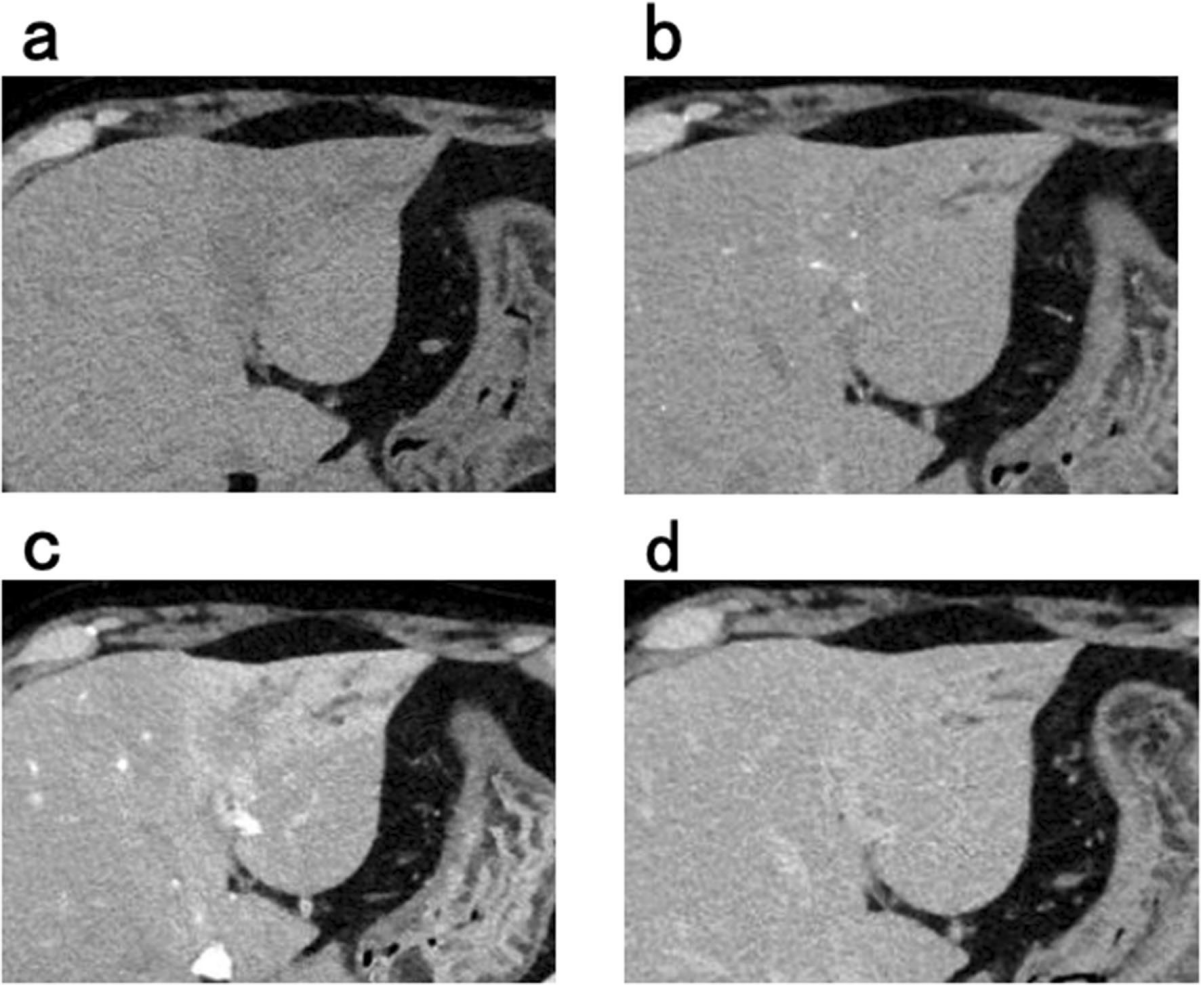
Abdominal enhanced computed tomography image showing a demonstrated an ambiguous lesion in S3 of the liver and dilatation of the intrahepatic and peripheral bile ducts. The lesion was poorly enhanced on b early and c portal phase, and d showed delayed enhancement on late phase
